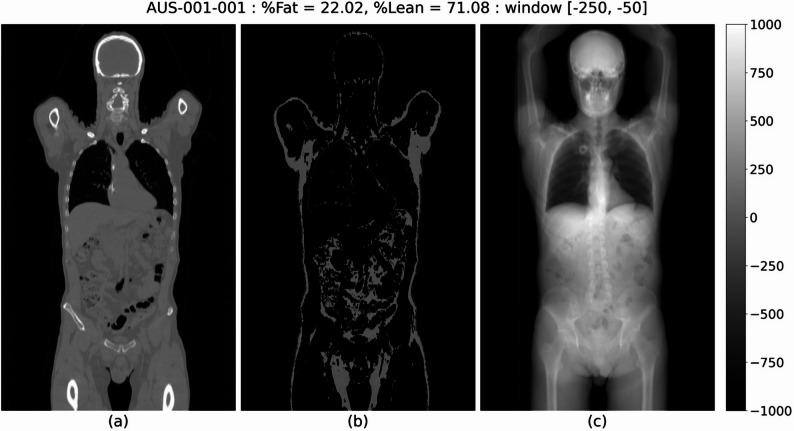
Low-dose CT of subject AUS-001, with a) whole-body tissue threshold (-300 HU); b) fat window (from − 250 HU to -50 HU); c) maximum intensity projection (MIP)
